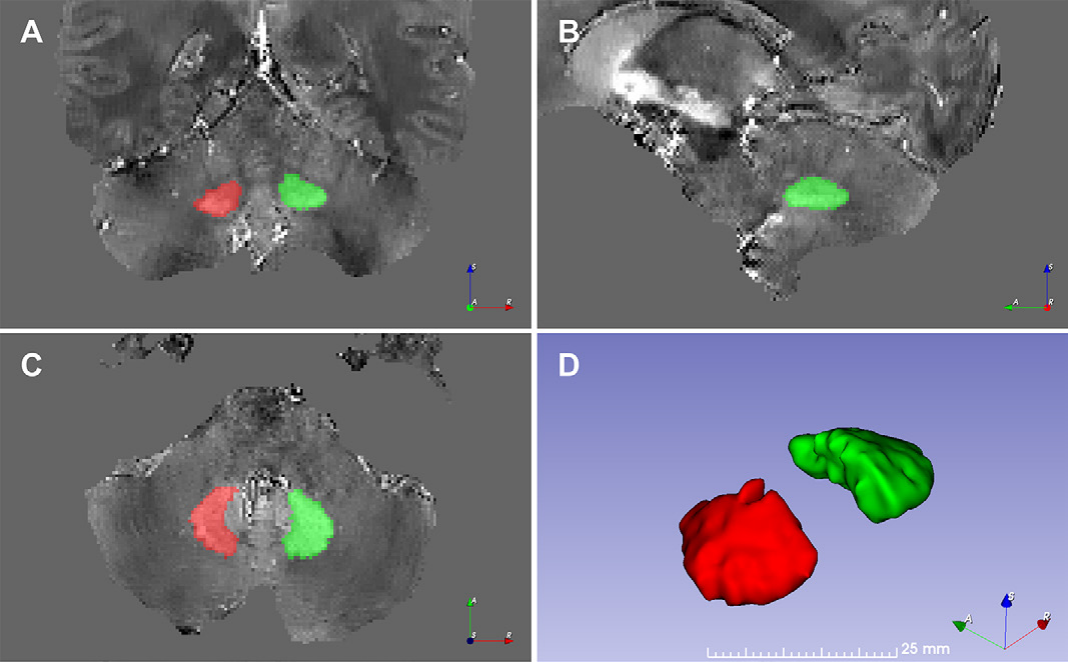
Manually segmented mask MR images in a 41-year-old female healthy control
